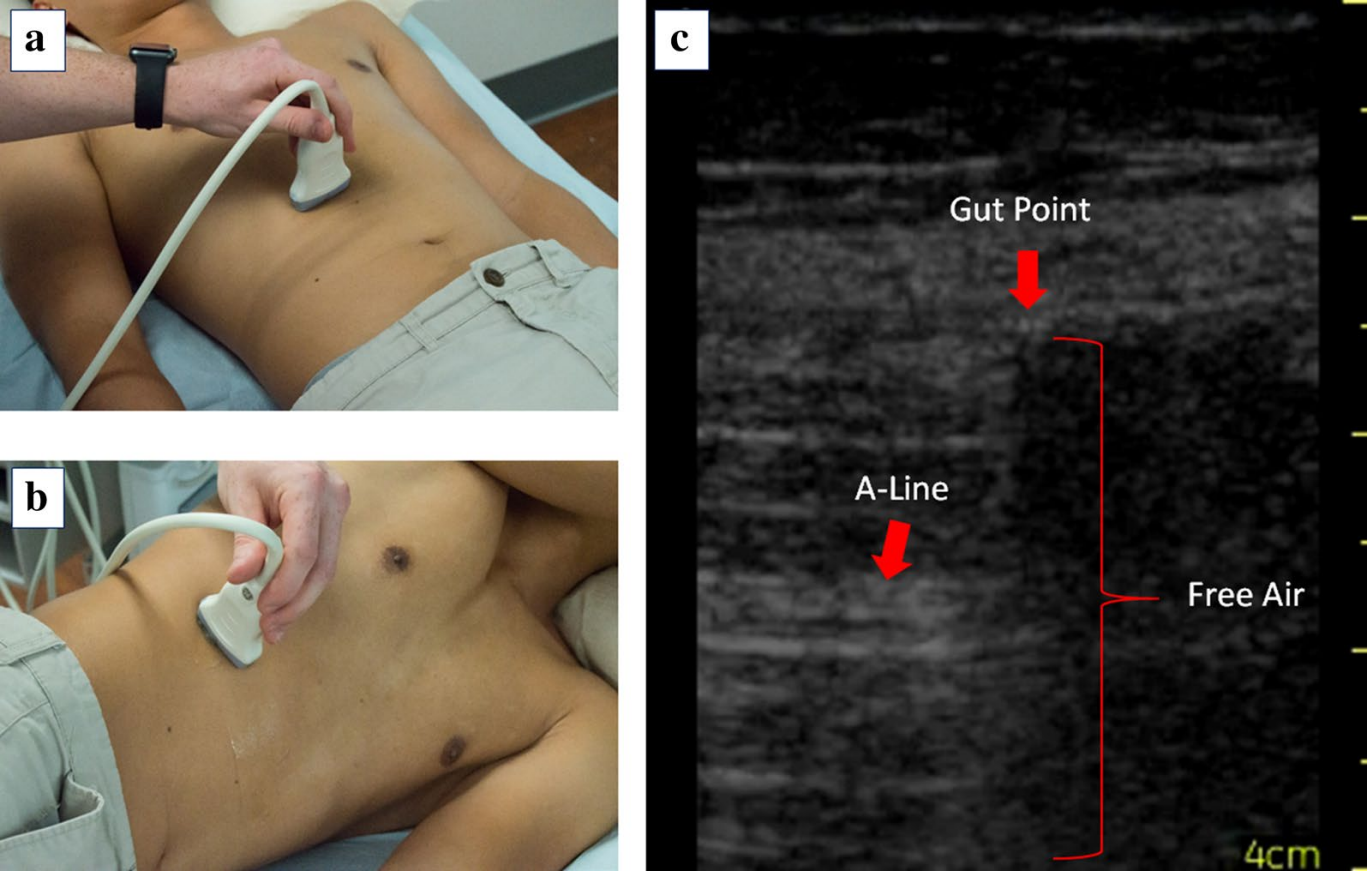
a Patient placed in the supine position for an initial sonographic assessment for free intraperitoneal air. b Patient placed in the lateral decubitus position to allow free intraperitoneal air to accumulate anterior to the hepatic parenchyma. c Sonographic output of a patient with free intraperitoneal air. Air is a strong reflector of ultrasound waves. Reflection produces bright focal lines (A-lines, red arrow) with dark shadowing in between
